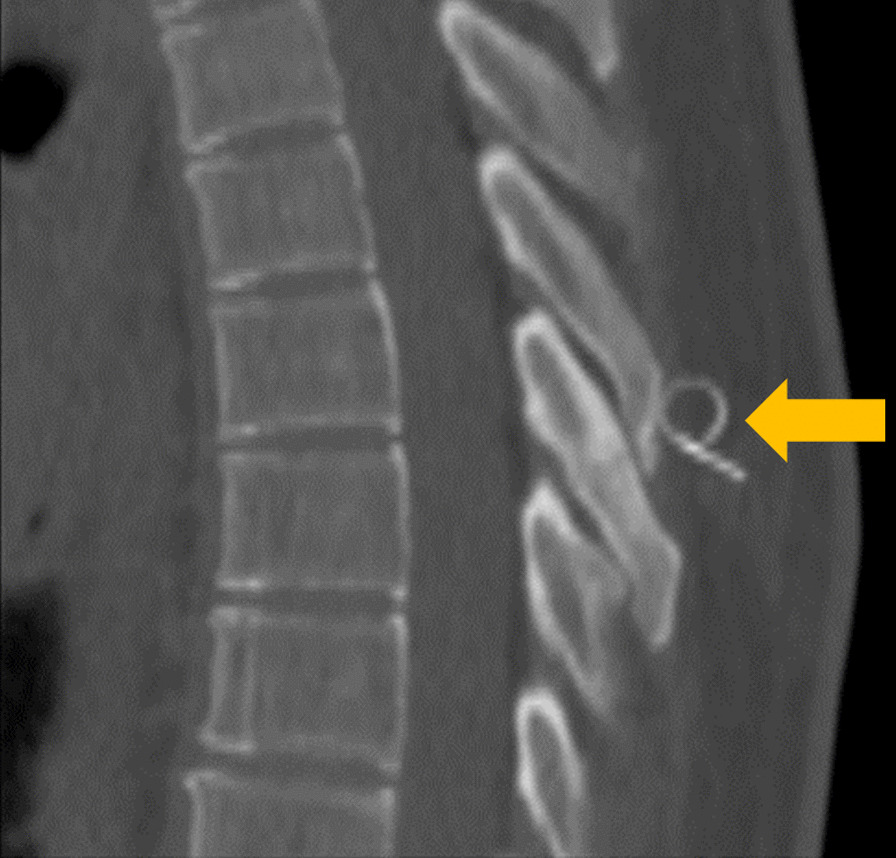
Pre-operative computed tomography with a landmark wire (gold arrow) to ensure the correct vertebral level
